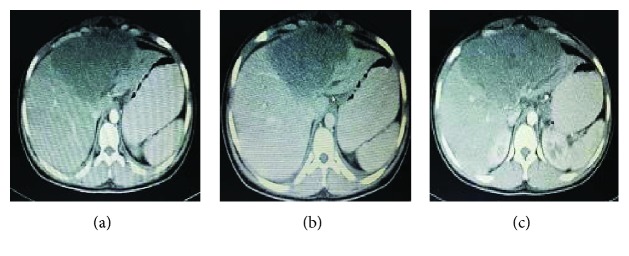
Contrast-enhanced CT abdomen. (a) Early arterial phase showing a hypodense lesion in the left liver lobe and a normal spleen. (b) Venous phase showing a hypodense lesion confined to the left liver lobe and firmly attached to the middle hepatic vein. (c) A mixed-enhanced pattern of the lesion on the delayed phase.
